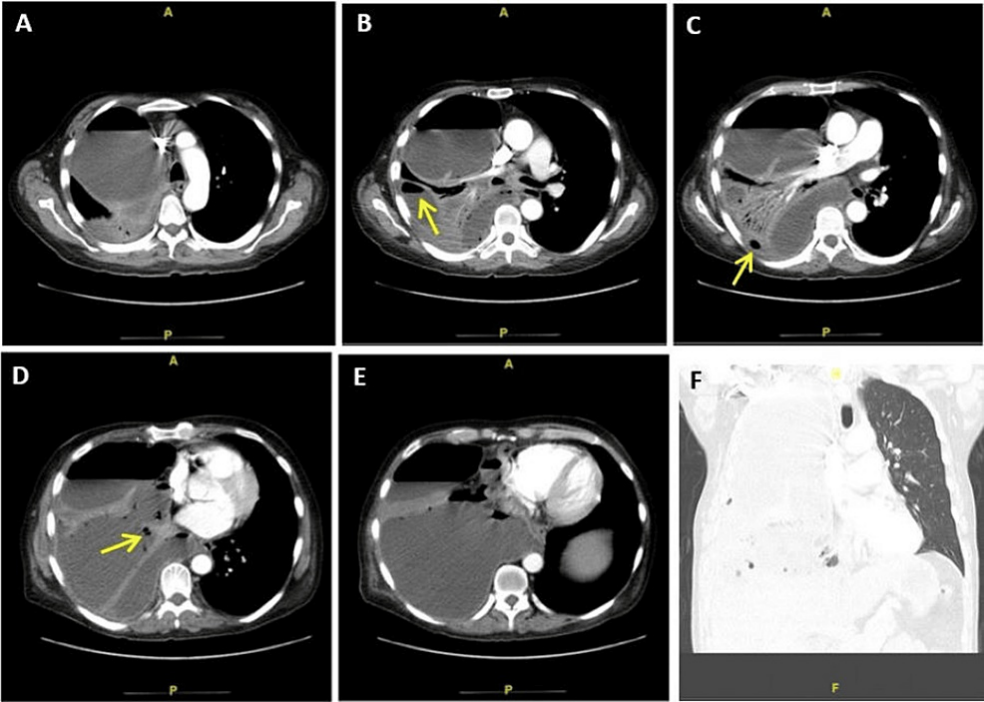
Images from CT thorax with contrast Images A to E: Anteroposterior (AP) view; Image F: Coronal view Note the air pockets within the fluid collection
